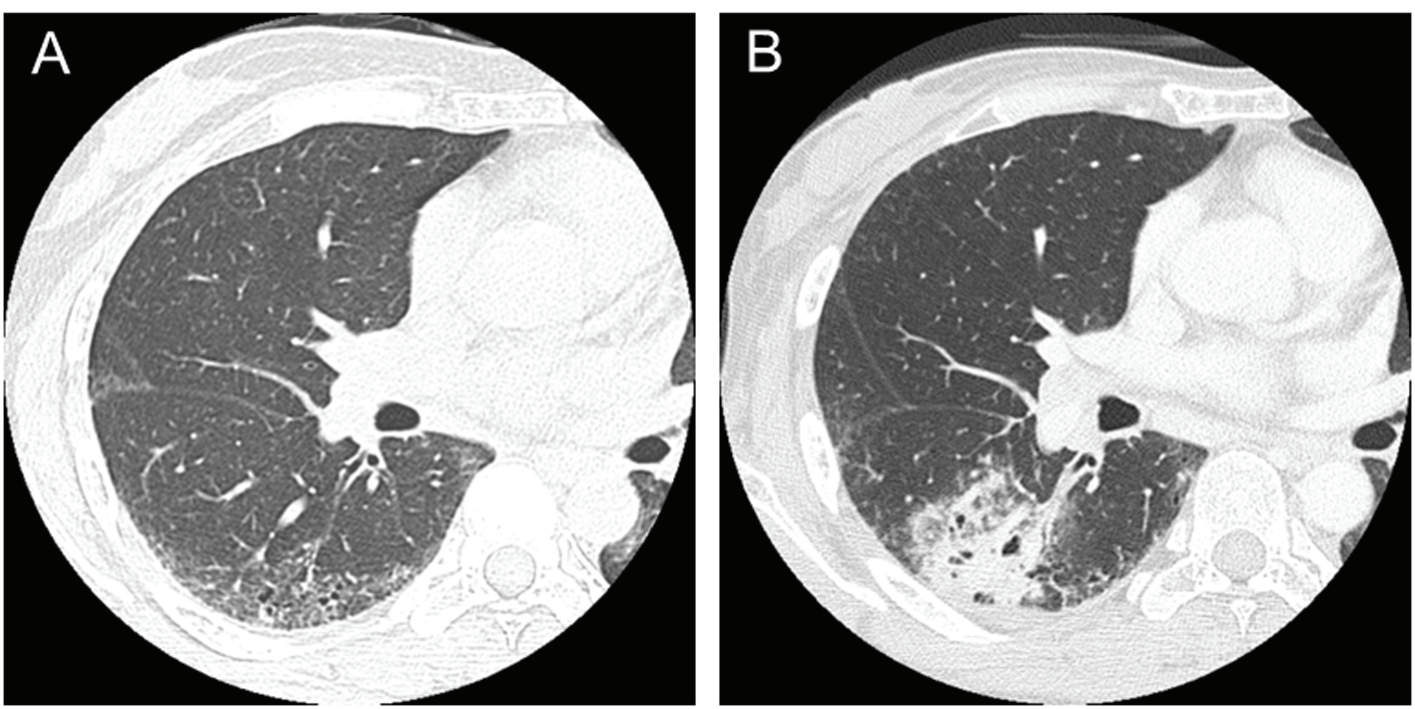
CT scans in a patient with systemic sclerosis. (A) Interstitial lung disease was observed in lower lobes in a 47-year-old patient. (B) Squamous cell lung cancer occurred in the area of interstitial lung disease in a 50-year-old patient.
